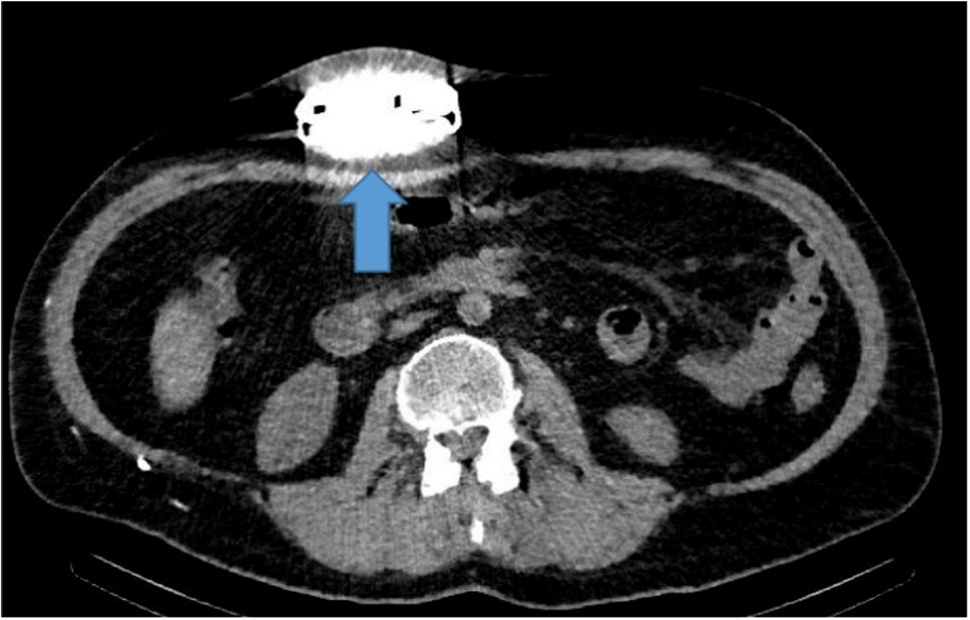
Edema (blue arrow) and suspected fluid collection on CT
